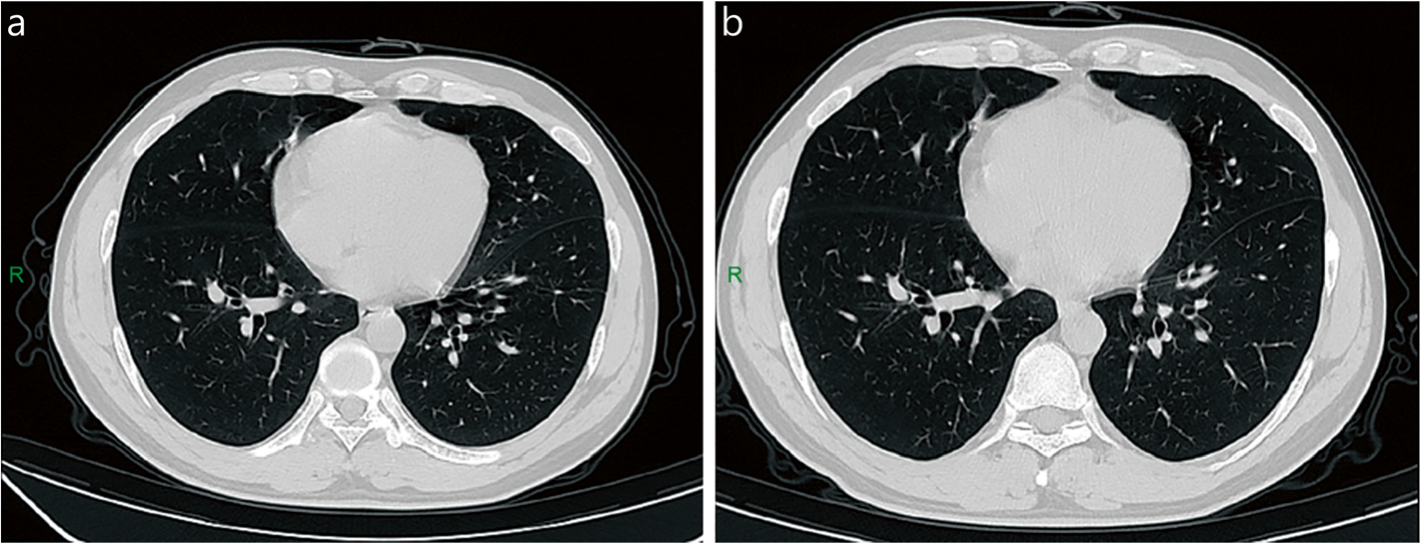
Chest CT of mild patient. A 27-year-old male patient was positive for SARS-CoV-2 after contact with COVID-19 and occasionally had a dry cough. A few old fibroses was seen in the middle lobe of the right lung, and there were no obvious changes in CT images on admission (a) and discharge (b). R: right
